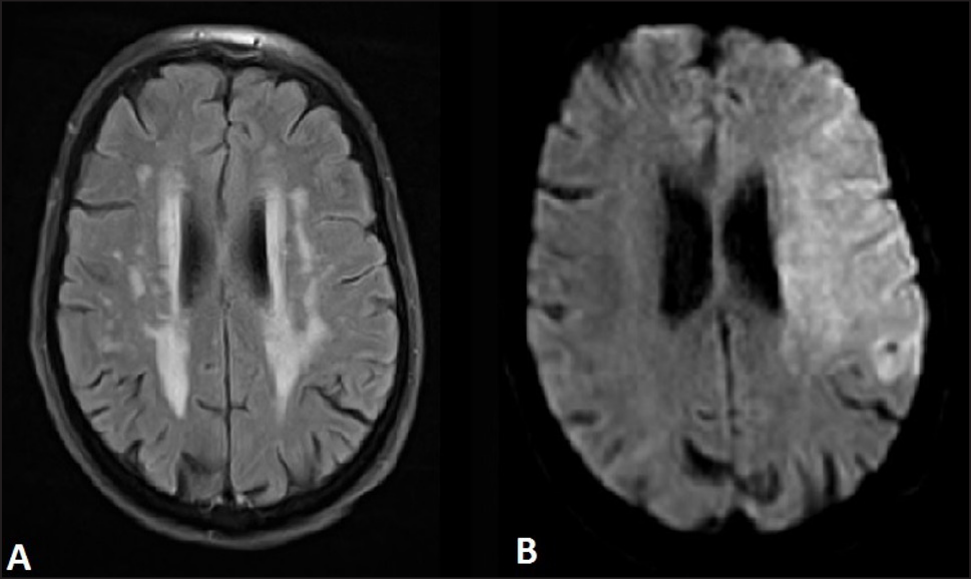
MRI of the brain with (A) axial FLAIR image showing leukoaraiosis and (B) axial DWI showing high signal intensity in the territory of left MCA.
